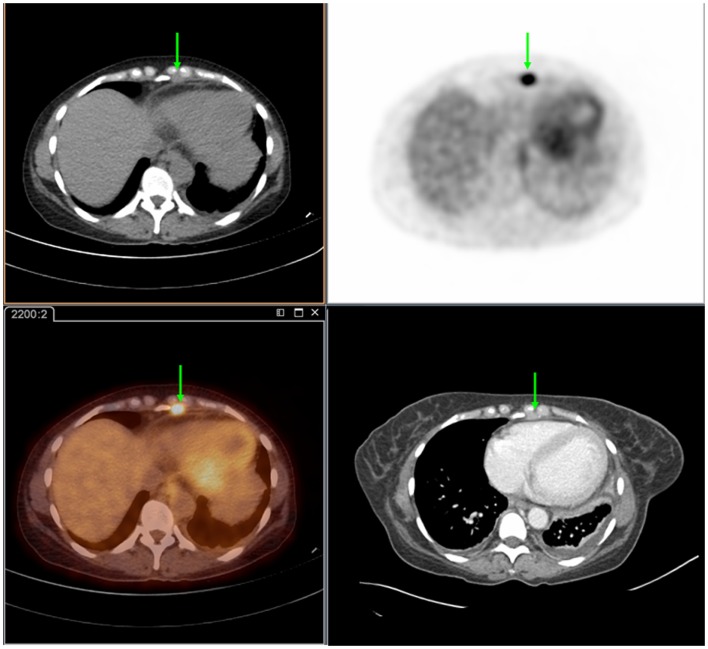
Seventy-two year-old female presenting with metastatic pancreatic adenocarcinoma. PET/CT demonstrates an FDG-avid cardiophrenic lymph node on non-contrast CT (top left), PET (top right), and fused images (bottom left). These were not commented on in the contrast-enhanced CT (bottom right). Differences in the levels between the two studies are due to patient positioning and respiratory motion.
